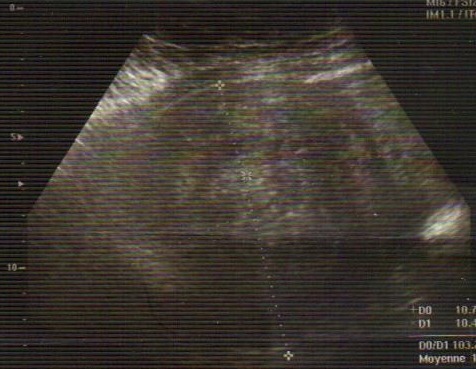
Ultrasound showing 11x10 cm serosal-type fundal uterine myoma
